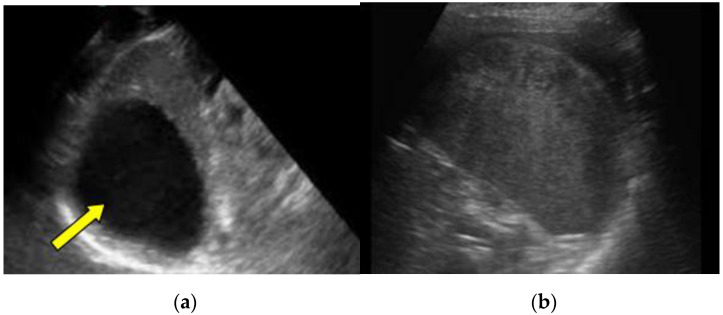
(a) A follow-up ultrasound examination, three months after the grade IV splenic injury (patient No. 16 in Table 3). Oblique ultrasound scans diagnosed regularly marginated hypoechoic pseudocyst measuring 50 × 55 mm^2^ in size; (b) A transverse scans on a follow-up ultrasound examination, 30 days after trauma revealed subcapsular hematoma 52 × 23 mm^2^ in size. Patient had grade III splenic injury after a fall from a standing height (patient No. 3 in Table 3).
